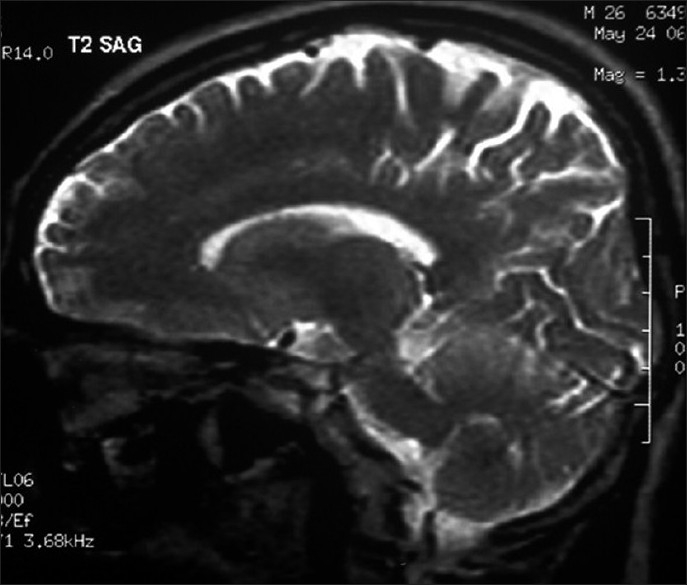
Normal T2 weighted saggital MRI brain in 2006
